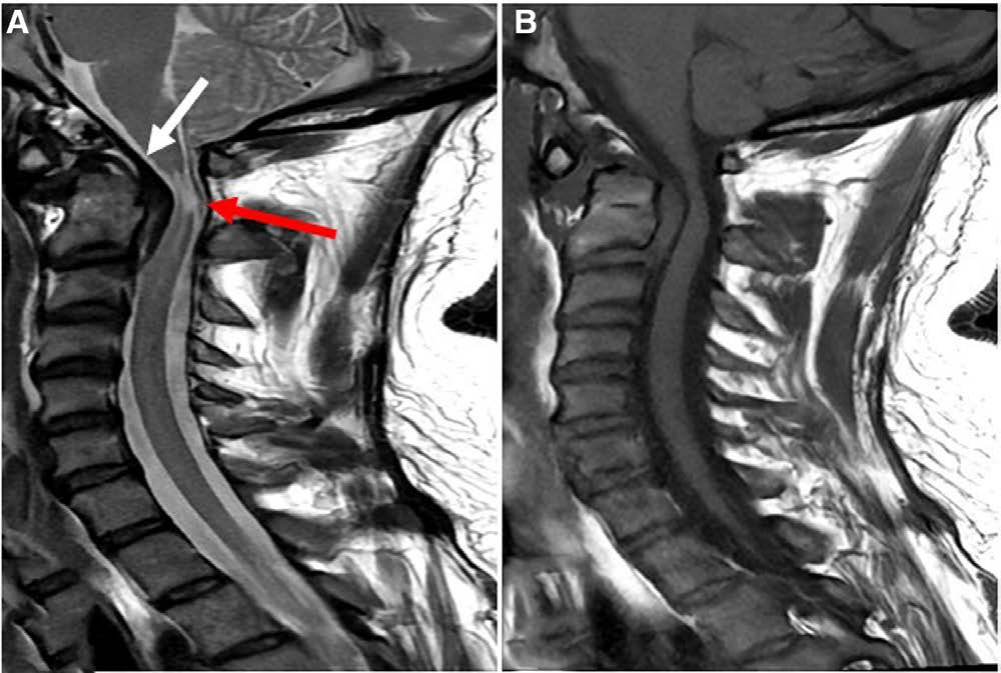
Imaging findings of a missed pretectal membrane hematoma in a 36-year-old male individual. Sagittal T2-weighted image (A) and sagittal T1-weighted image (B) fast spin echo show spinal cord myelomalacia (red arrow) due to a large old hematoma located deep to the tectorial membrane (white arrow). The hematoma demonstrates mildly hyperintense internal content with a peripheral low signal border on the T2-weighted image and iso-signal appearance on the T1-weighted image relative to the cord’s white matter.
